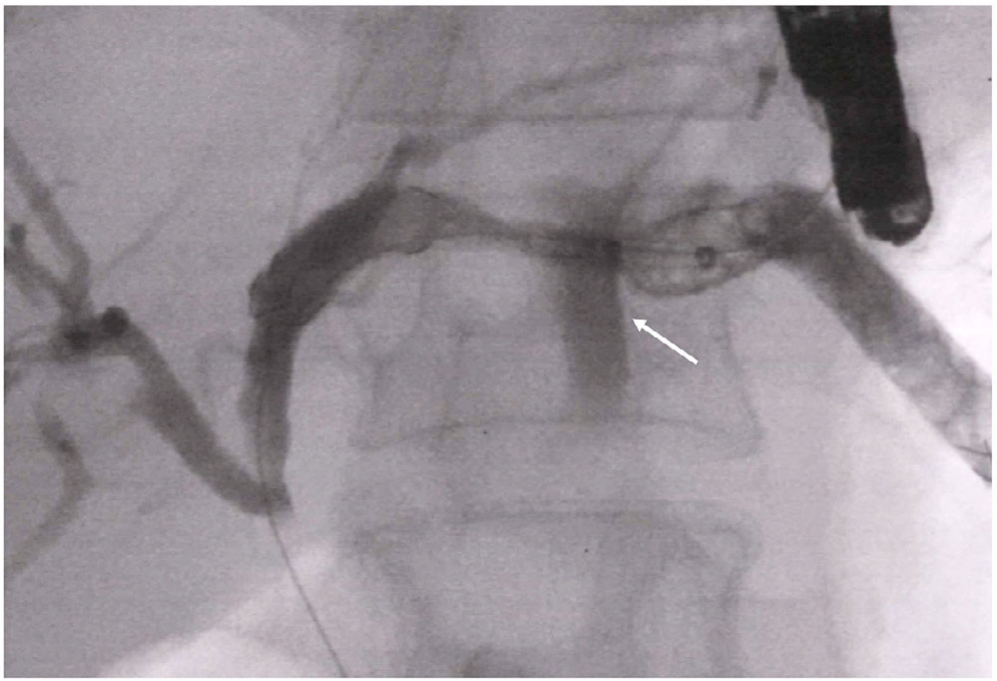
Bile leakage due to damage to the cover membrane of an HGS stent. This case involved EUS‐HGS with a partially covered self‐expandable metal stent. The day after the procedure, the patient complained of abdominal pain and computed tomography revealed fluid collection around the stomach and spleen. Balloon‐occluded cholangiography demonstrated contrast leakage from the HGS stent between the stomach and the liver (→), necessitating the placement of an additional covered metal stent inside the HGS stent to seal the leak. EUS‐HGS, endoscopic ultrasound‐guided hepaticogastrostomy.
